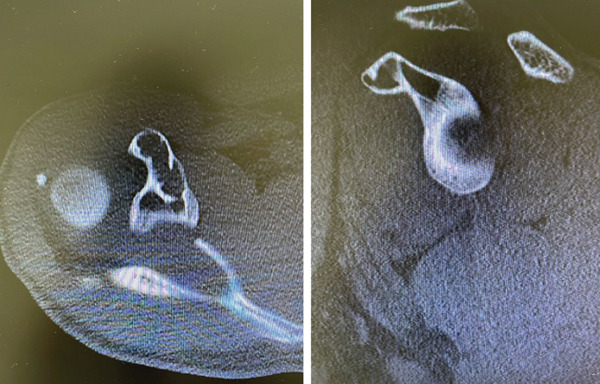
Preoperative computed tomography (CT) scan of the right shoulder demonstrating a well‐defined, expansile osteolytic lesion located in the coracoid process. The lesion presents with cortical thinning and multiloculated appearance, without evidence of cortical breach or periosteal reaction. These features are consistent with an aneurysmal bone cyst.
